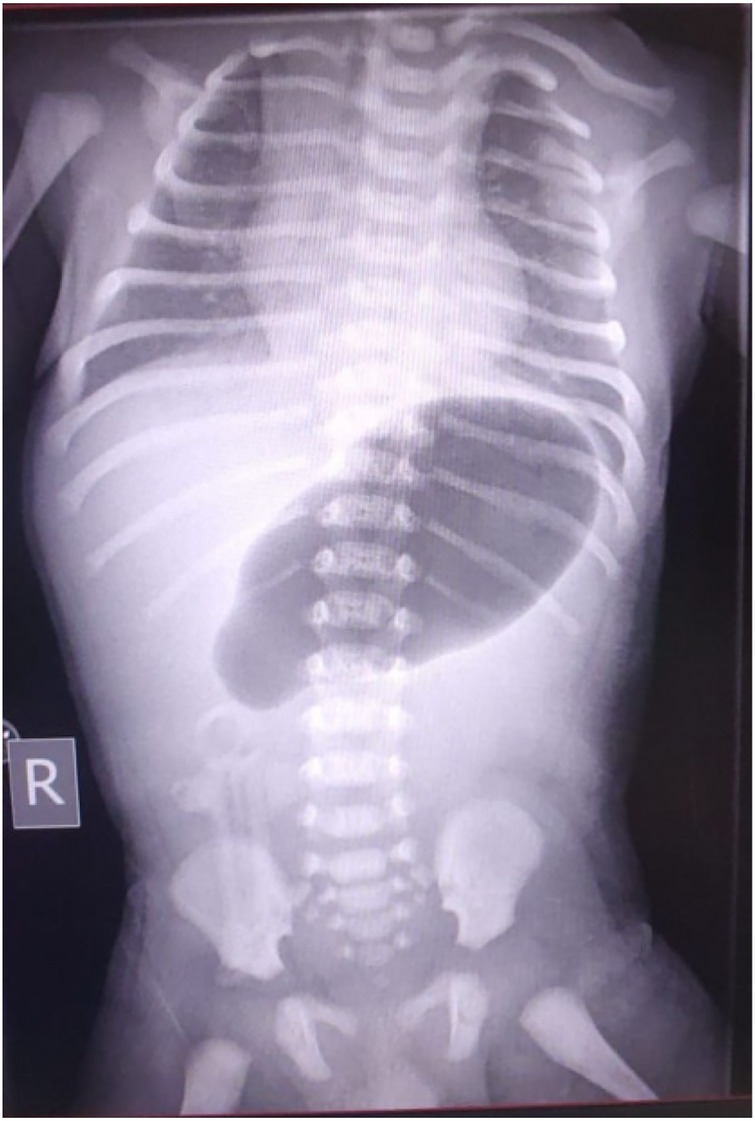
An Abdominal x-ray showing a single bubble sign consistent with pyloric atresia.
